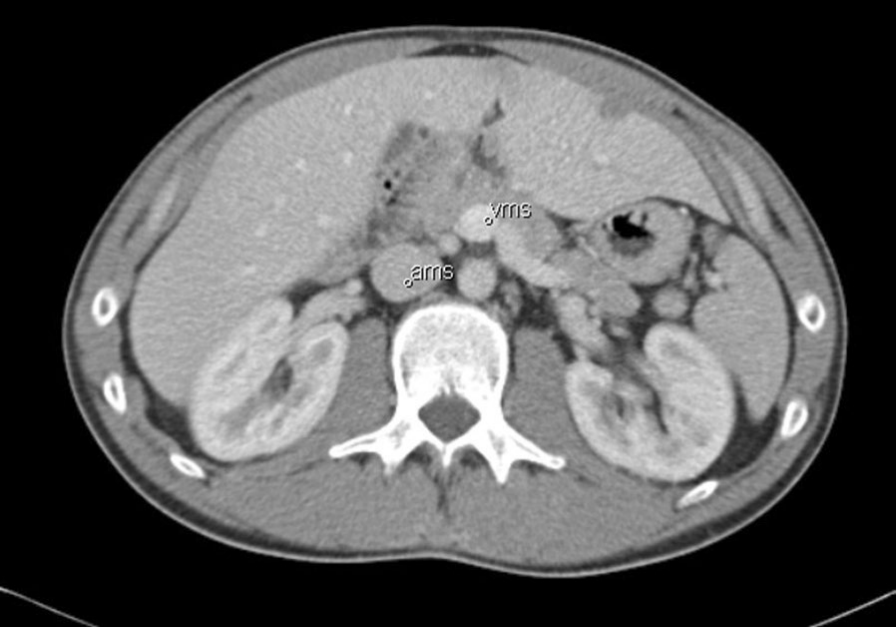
CT scan in axial position showing the inverted vessels
